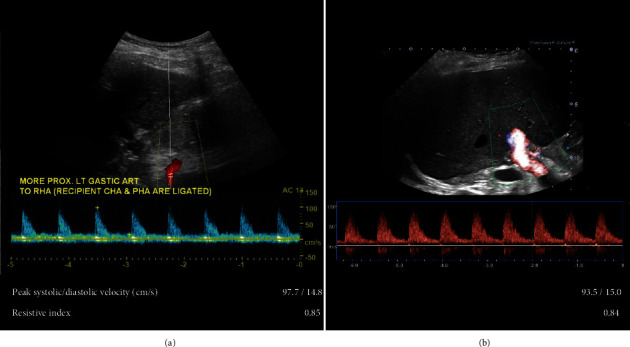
Case 2 Doppler ultrasound (US) imaging on postoperative day 1 with appropriate waveforms at the alternatively reconstructed anastomosis with inflow from the left gastric artery (a). Repeat US nine-month posttransplant with stable findings (b).
